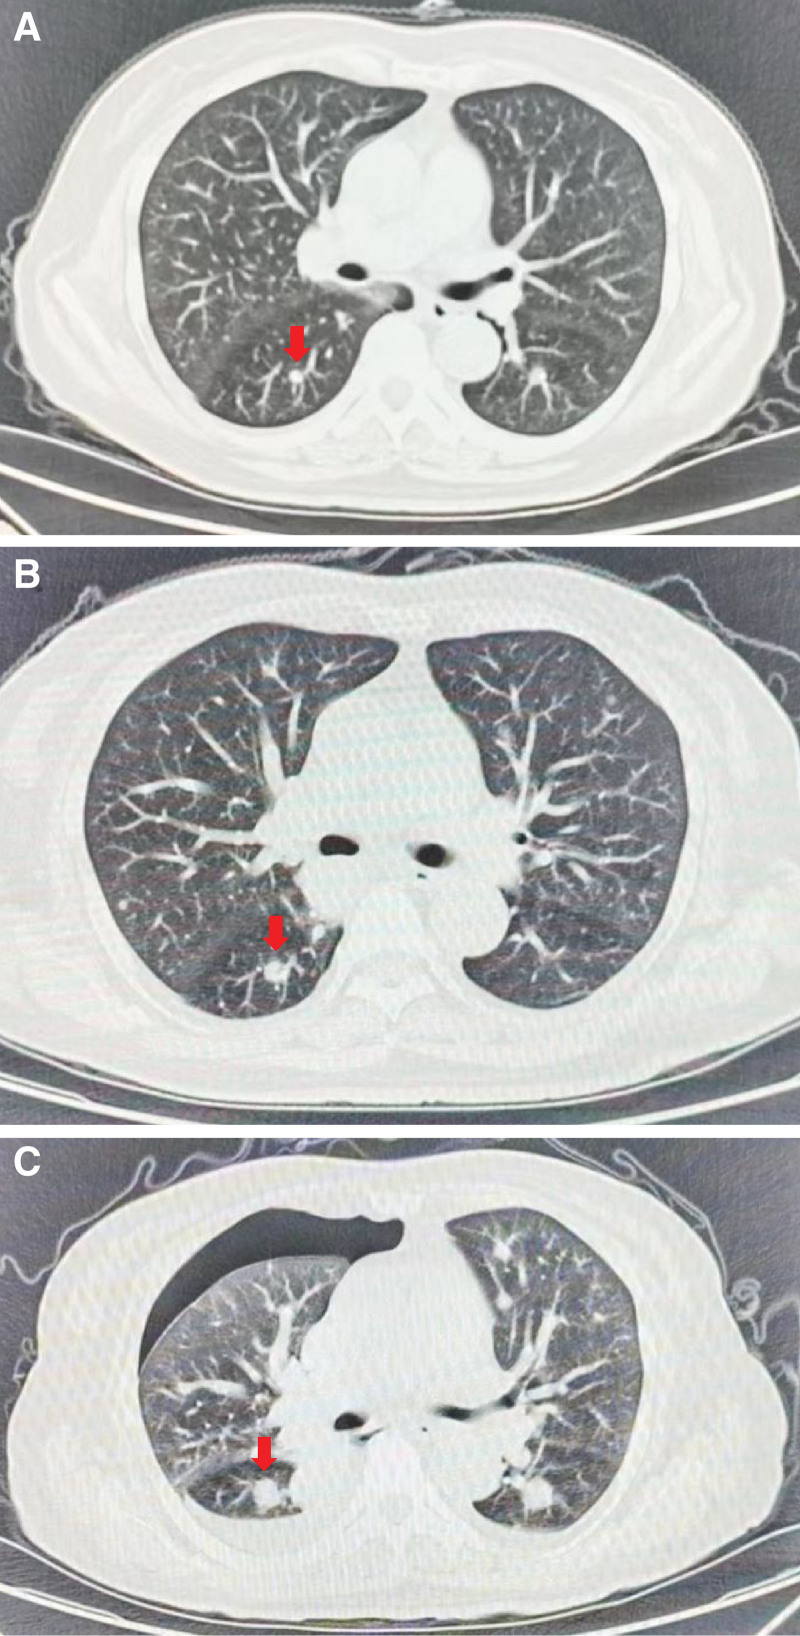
Computed tomography (A–C) shows multiple solid-density nodular shadows in both lungs, with the larger ones located in the dorsal segment of the right lower lobe and progressively enlarged (red arrow). (A) (2 days before surgery): the size of the pulmonary nodule is approximately 16 × 14 mm (Se203im28), and the boundary remains clear. (B) (11 days after surgery): the size of the pulmonary nodule is approximately 25 × 23 mm (Se203im23), and the boundary remains clear. (C) (24 days after surgery): the size of the pulmonary nodule is approximately 34 × 24 mm (Se203im24), and the boundary remains clear.
